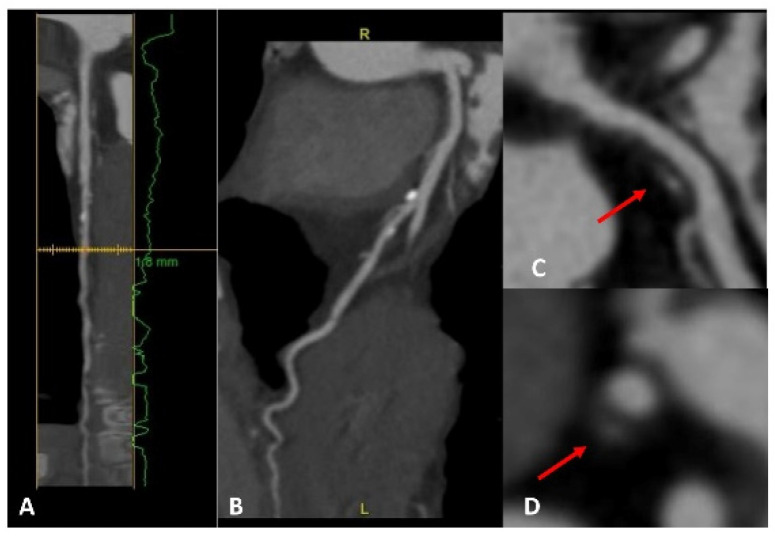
A patient with a strong family history of CAD, with cardiac CT positive for non-obstructive CAD on proximal LAD (panels A,B). A high-risk fibrolipidic plaque (red arrow) with positive remodeling, low attenuation, and small spotty calcification is evident in both the long (panel C) and short (panel D) axis views.
